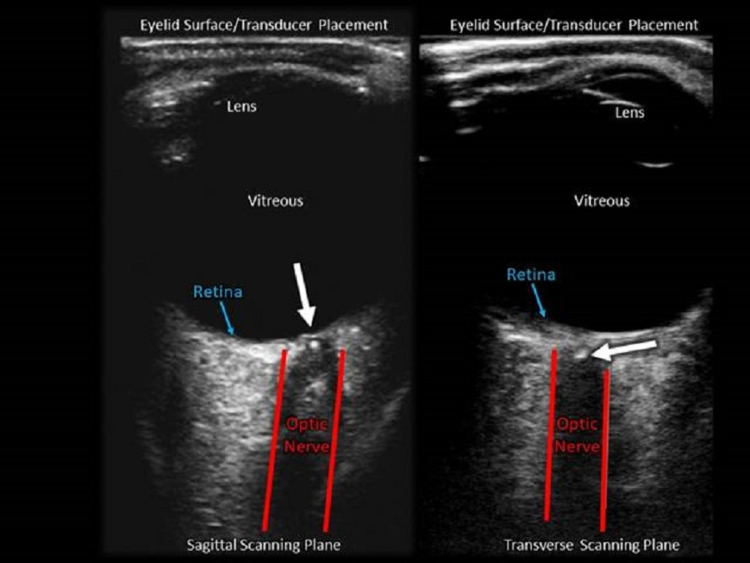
Sagittal and transverse scanning plane in an ocular point-of-care ultrasound showing "spot sign".
